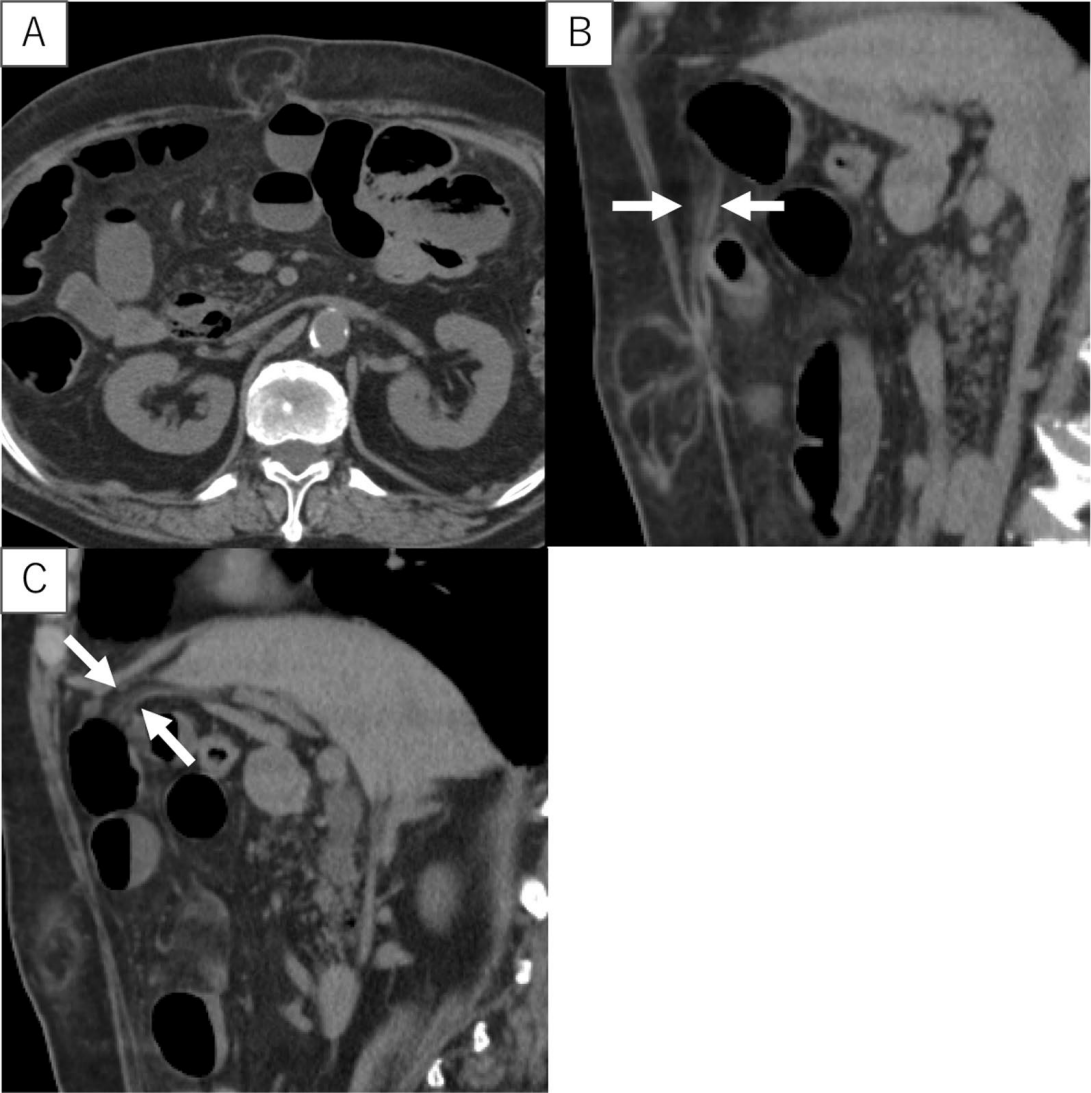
Computed tomography scan. A Adipose tissue herniated from the linea alba. B, C Adipose tissue contiguous with the hepatic round ligament (white arrow)
